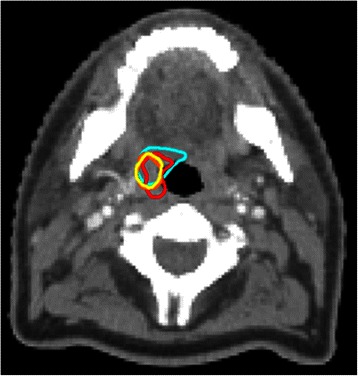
Representative example of GTVs delineated on CT, MR, CT-MR by a single radiation oncologist, displayed on an axial CT scan, for a patient with a T1 N2b well differentiated squamous cell carcinoma of the right tonsil. CT GTV red; MR GTV yellow; CT-MR contour orange; PET contour blue
